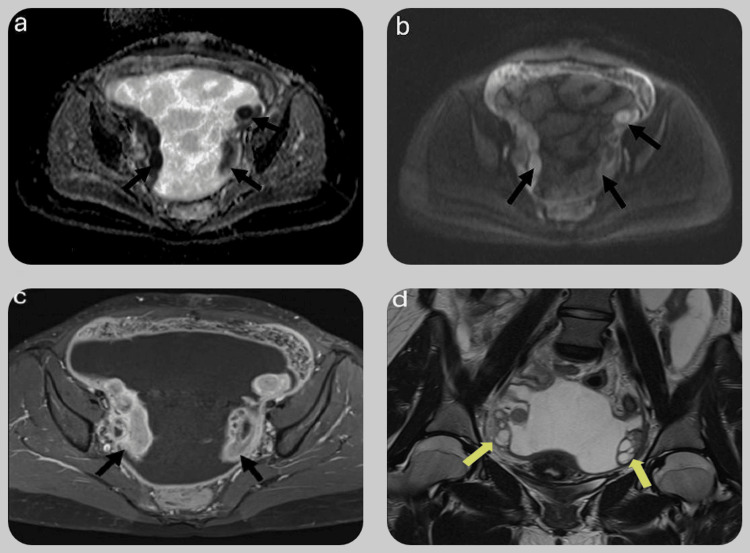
Pelvic MRI MRI pelvis images demonstrating three sequences: diffusion-weighted imaging (DWI) (a), apparent diffusion coefficient (ADC) (b), and post-contrast sequence (c). These images show intense mucosal enhancement of both fallopian tubes with restricted diffusion (black arrows), consistent with bilateral salpingitis. (d) Coronal MRI section showing normal bilateral ovaries (yellow arrows).
